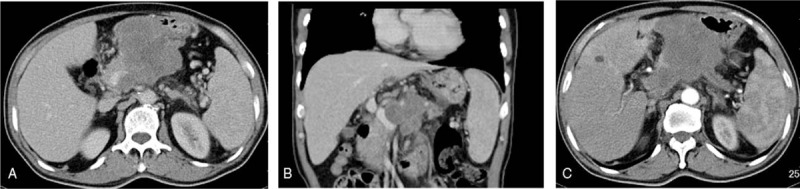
Preoperative CT images of the patient. A. CT revealed a large mass located at the pancreatic body. B. The portal vein was invaded by the tumor. C. The main pancreatic duct was dilated to 6.5 mm. CT = computed tomography.
